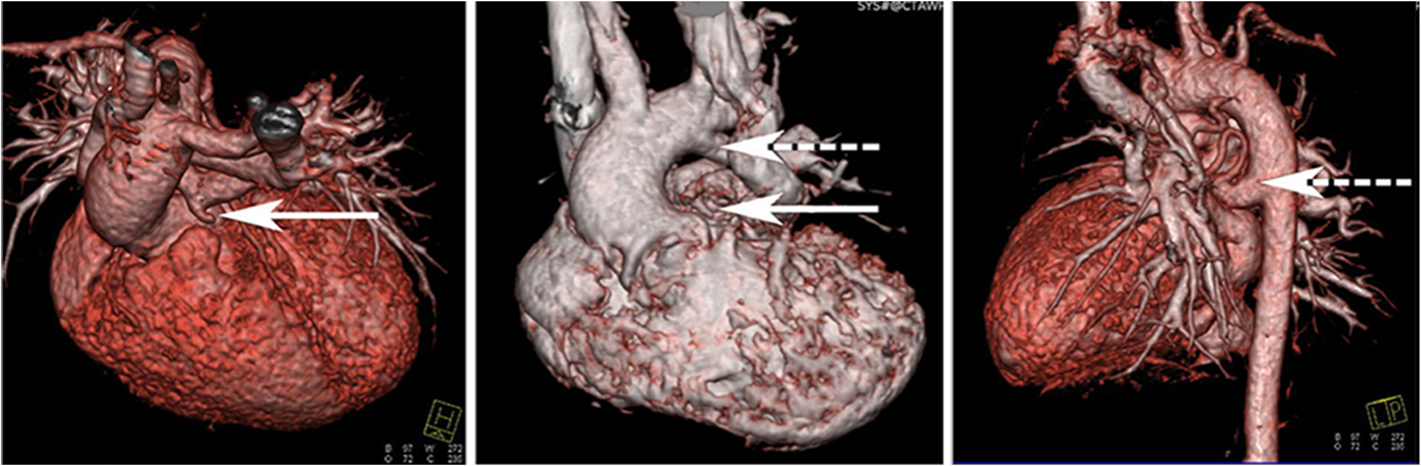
Computed tomography angiography pre-surgery. *Block arrows highlight* the pulmonary atresia, and the *dashed arrows highlight* the patent ductus arteriosus (PDA) or the major aortopulmonary collateral arteries (MAPCA)
